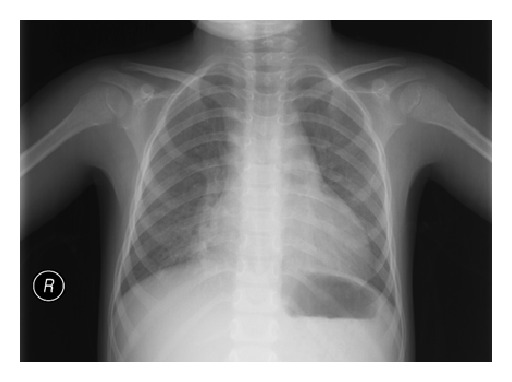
Chest X-ray demonstrating interstitial infiltration.
